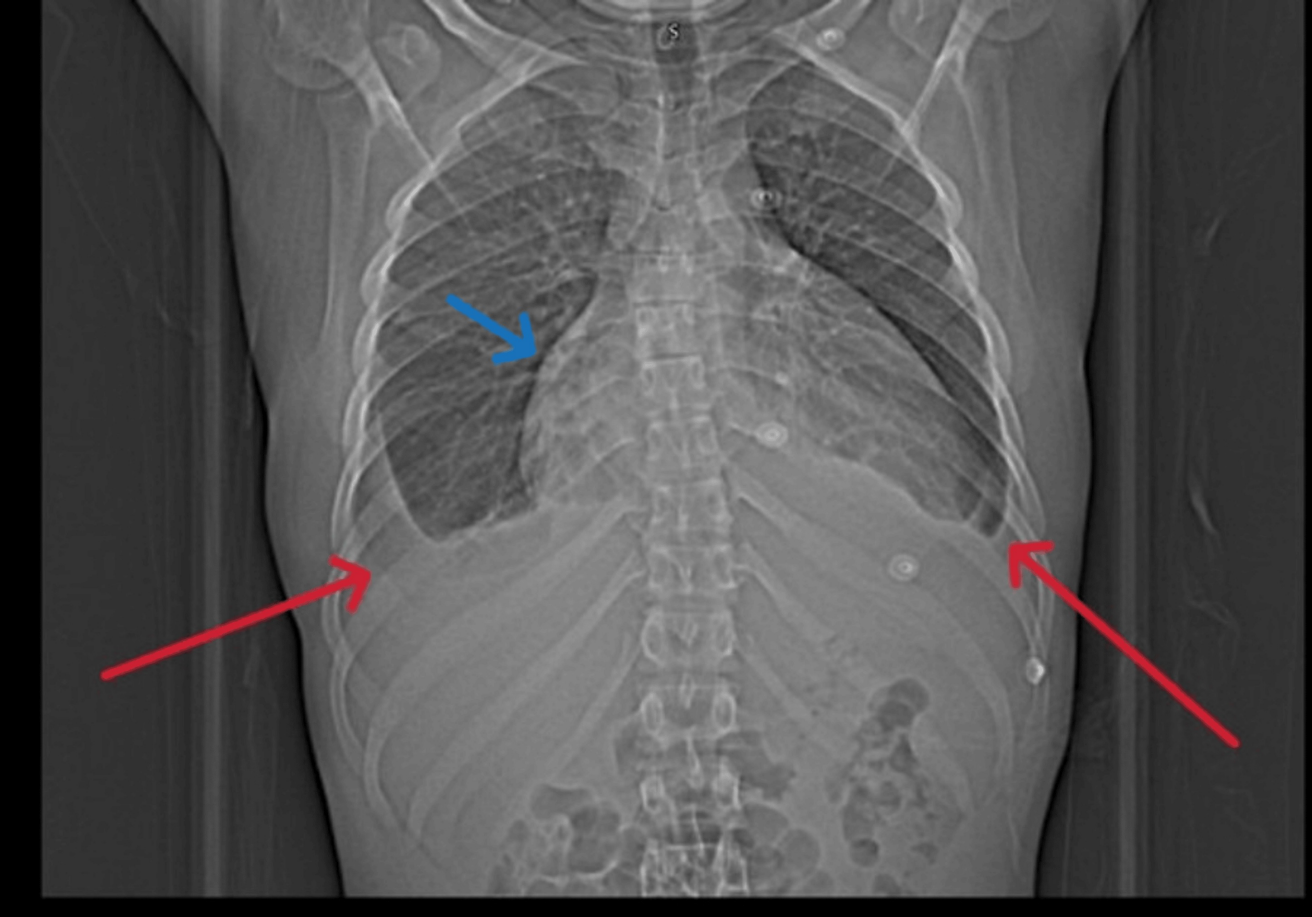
Chest X-ray (PA view) The red arrows showed bilateral pleural effusion, the blue arrow revealed cardiomegaly with pericardial effusion. PA: posteroanterior
